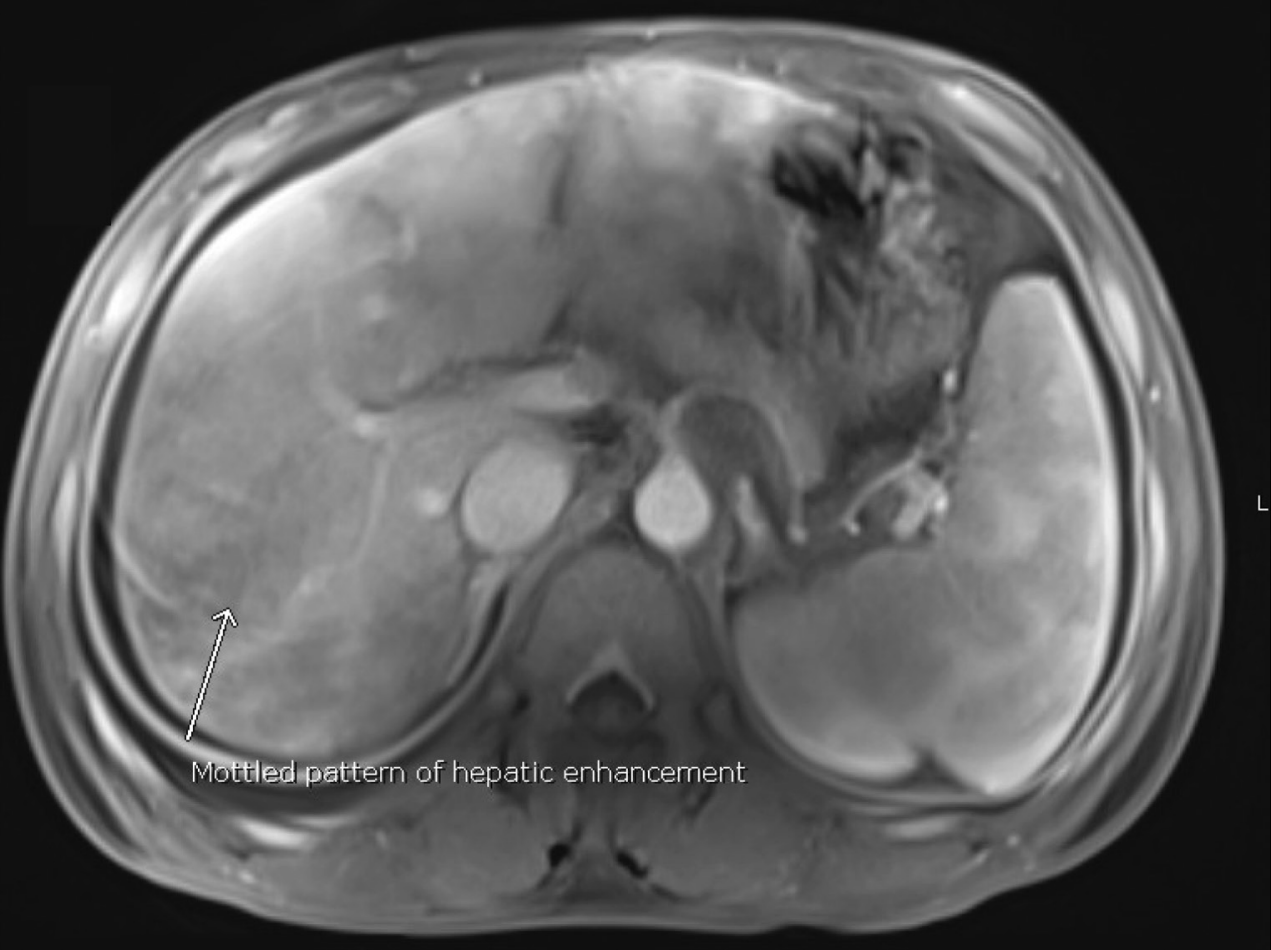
MRI abdomen with intravenous contrast showing mottled appearance of the liver parenchyma.
